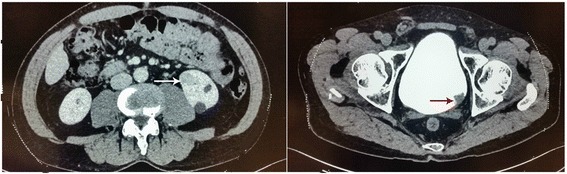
CT of a patient with concurrent renal cell carcinoma and bladder tumor. The *white arrow* shows a renal mass in the left kidney; the *red arrow* shows a filling defect in the left posterior wall of bladder
